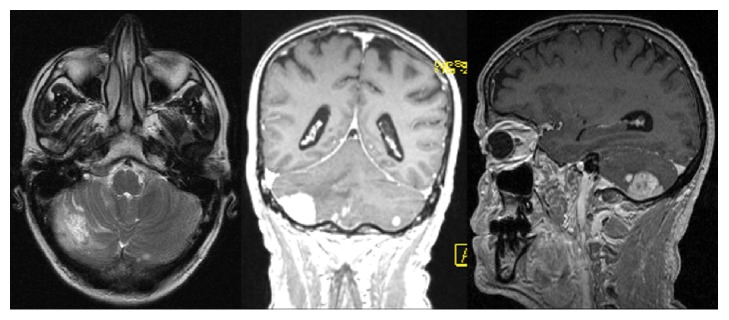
Magnetic resonance imaging showing multiple bilateral lesions in the cerebellum with strong and irregular contrast enhancement, central necrosis, and mass effect.
